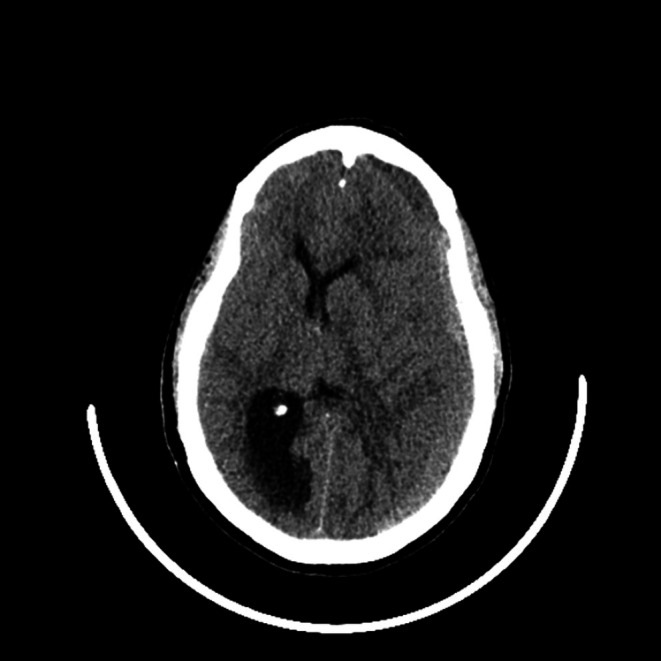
Pre‐operative CT axial scan demonstrating a large, holohemispheric, mixed density extra‐axial fluid collection with associated significant mass effect, midline shift and left‐sided uncal herniation.
